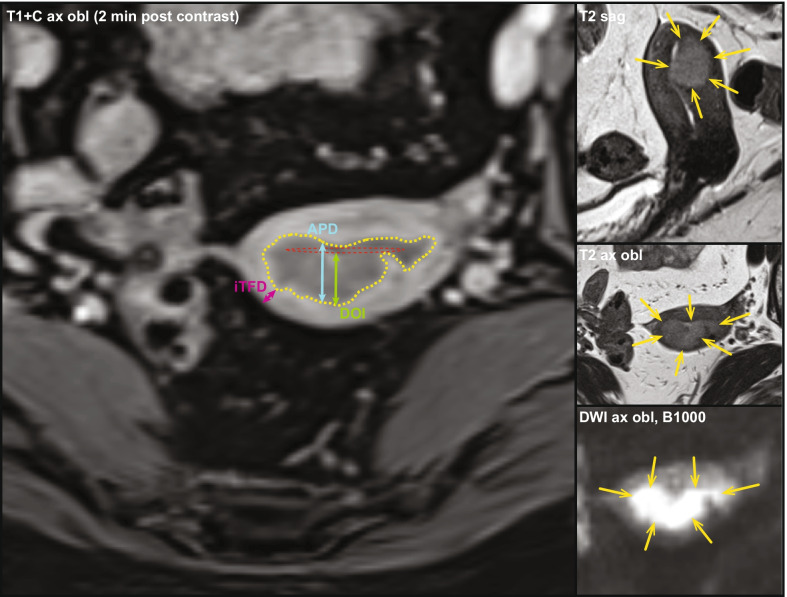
Pelvic 3 T MRI of a 68-year-old patient with endometrial cancer, FIGO stage 1B (≥ 50% myometrial invasion). Tumor was MRI-staged to iDMI (≥ 50%), maximum anteroposterior tumor diameter (APD) 17 mm, maximum depth of myometrial invasion (DOI) 7 mm and tumor-free distance to serosa (iTFD) 5 mm. The different MRI measurements are marked on an axial oblique contrast-enhanced T1-weighted image, 2 min after contrast injection. The measurements are supported by sagittal and axial oblique T2-weighted images (T2 sag and T2 ax obl) and axial oblique diffusion-weighted B-1000 image depicting restricted diffusion in the tumor. Tumor is marked with yellow arrows and yellow dotted line. Red oval line = presumed lining of the uterine cavity, green arrows = DOI, pink arrows = iTFD and blue arrows = APD
